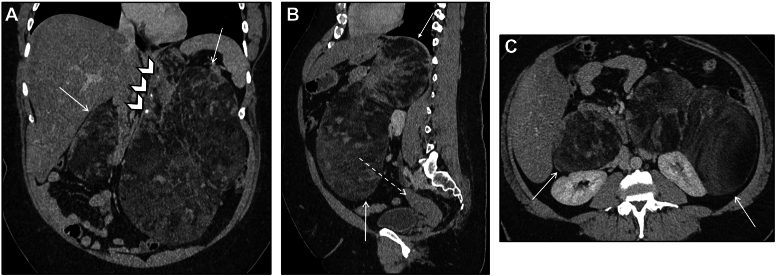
Bilateral adrenal myelolipomas in congenital adrenal hyperplasia. Coronal (*A*), sagittal (*B*), and axial (*C*) contrast-enhanced CT images on the 75 s delayed phase show bilateral large adrenal masses (arrows) with macroscopic fat and a few coarse calcifications (arrowheads) consistent with bilateral myelolipomas. Note this male patient has a uterus as they were assigned female at birth (dashed arrow). *CT* = computed tomography.
